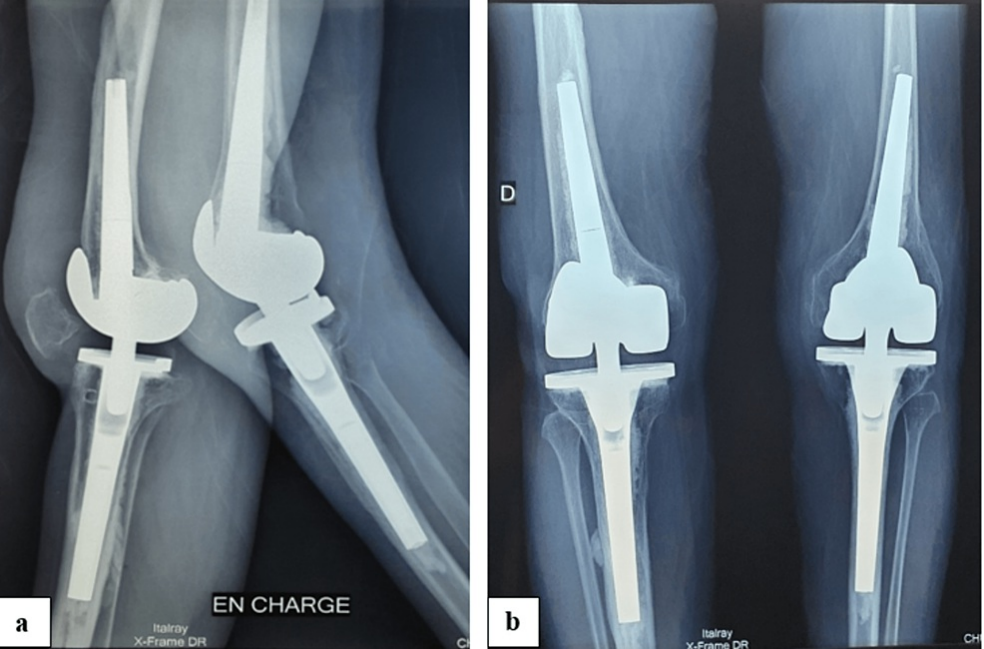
Lateral (a) and frontal (b) views of six months postoperative X-ray showing good alignment with no signs of loosening.
